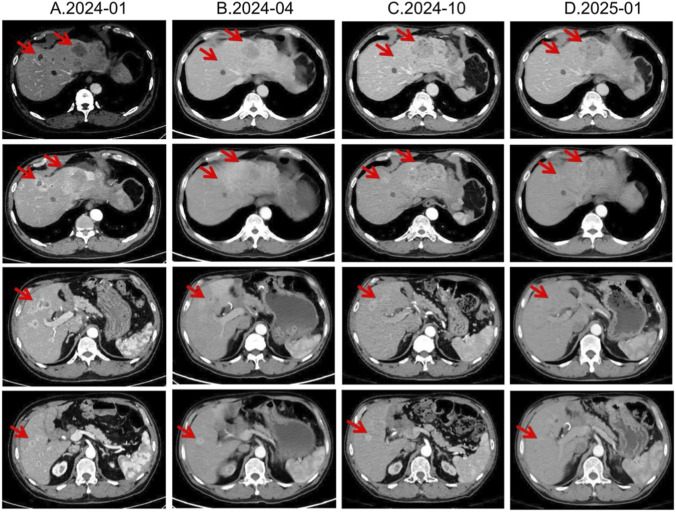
Lesion changes in patients during third-line everolimus treatment. (A) 2024-01 CT shows: Multiple weakly enhanced nodules in the liver, the largest of which is approximately 4.3 cm × 2.8 cm in size. (B) 2024-04 CT shows: The liver lesions have decreased and shrunk compared with most of the previous lesions. Treatment response evaluation: PR. (C) 2024-10 CT shows: Multiple slightly low-density nodular mass shadows in the liver, especially in the left outer lobe of the liver. Enhanced scans show uneven enhancement. Treatment response evaluation: SD. (D) 2025-01 CT shows: Multiple slightly low-density nodular mass shadows in the liver, especially in the left outer lobe, and enhanced scans show heterogeneous enhancement. Treatment response evaluation: SD.
